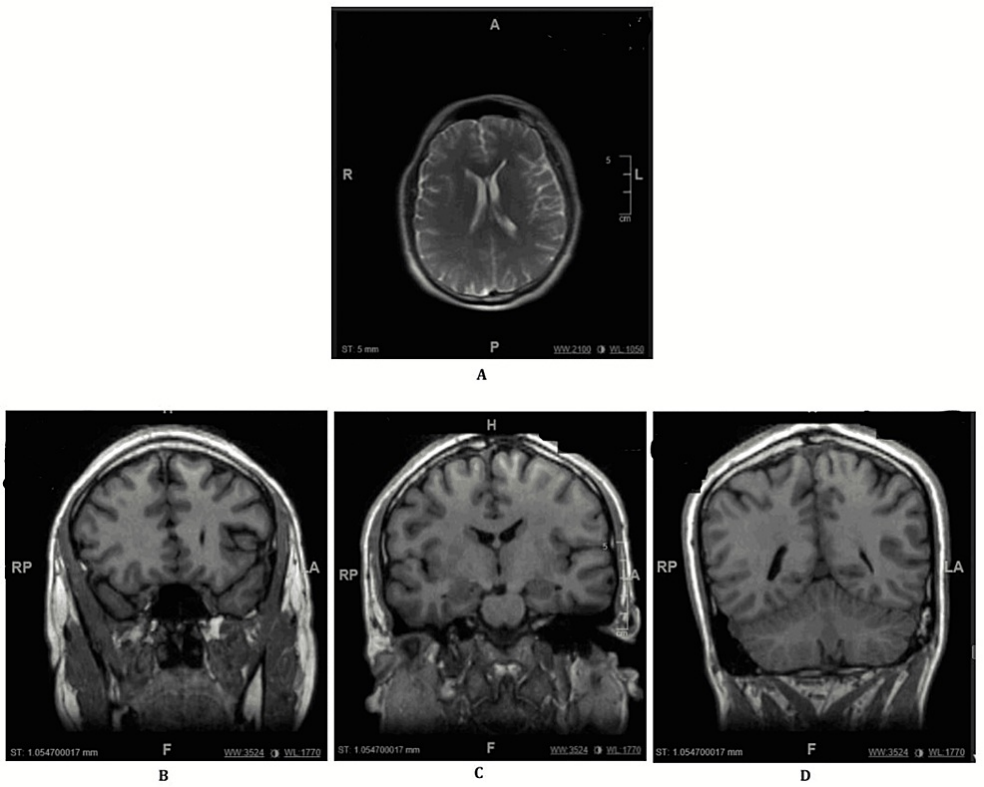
MRI images. Impression: No evidence of intracranial hemorrhage, mass, or infarct. No abnormal parenchymal or extra-axial enhancement. (A) Dorsal slice of the patient's brain. (B-D) From left to right, anterior to posterior coronal slice of the patient's brain.
